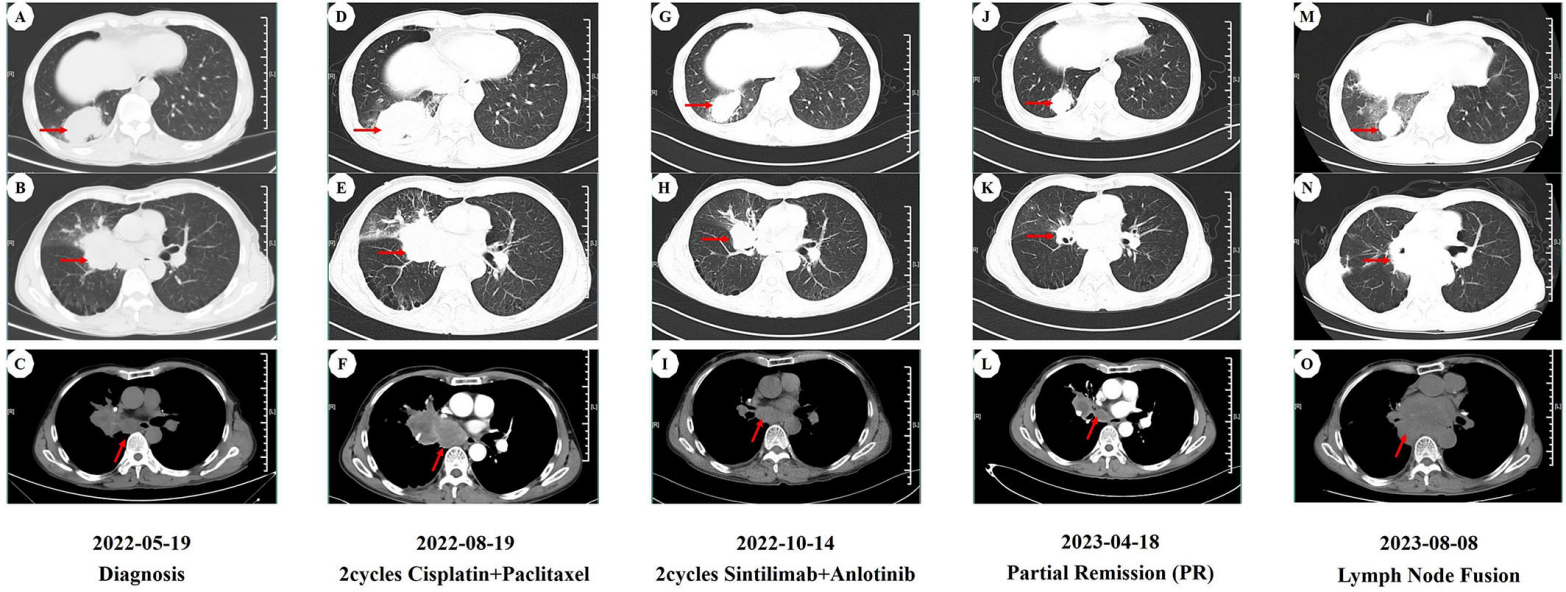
CT imaging assessment of lung lesions and mediastinal lymph nodes throughout the course of disease corresponding to their respective time points. (A–C) CT imaging of the lung window (A, B) and the mediastinal window (C) on May 19, 2022; (D–F) CT imaging of the lung window (D, E) and the mediastinal window (F) on August 19, 2022; (G–I) CT imaging of the lung window (G,H) and the mediastinal window (I) on October 14, 2022; (J–L) CT imaging of the lung window (J, K) and the mediastinal window (L) on April 18, 2023; (M–O) CT imaging of the lung window (M, N) and the mediastinal window (O) on August 8, 2023. (A, D, G, J, M) image manifests the lesion of the right lower lobe in the CT lung window. (B, E, H, K, N) image manifests the lesion of the right middle lobe in the CT lung window. (C, F, I, L, O) image manifests the mediastinal lymph nodes in the CT mediastinal window.
